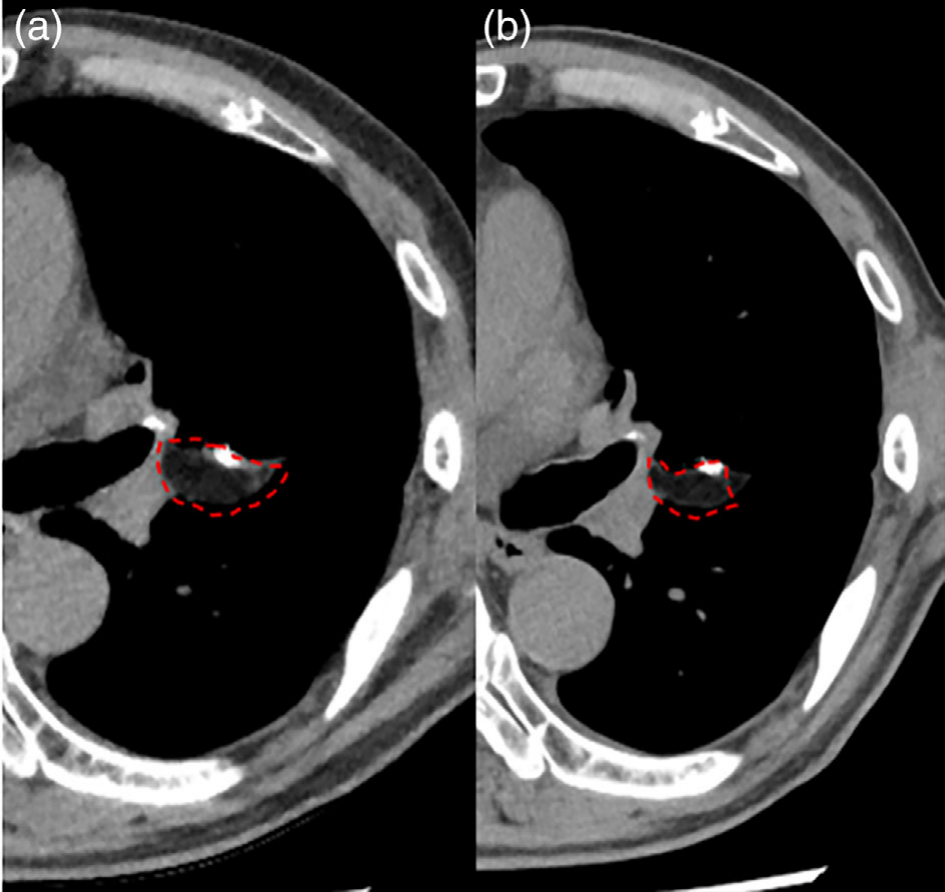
Images of the residual FPFP. (a) The residual FPFP (dotted line) of one of the patients was found on computed tomography (CT) scan undertaken 6 months after surgery. (b) The FPFP (dotted line) still remained on postoperative CT that was performed 1 year after surgery although it was slightly reduced. FPFP, free pericardial fat pad.
